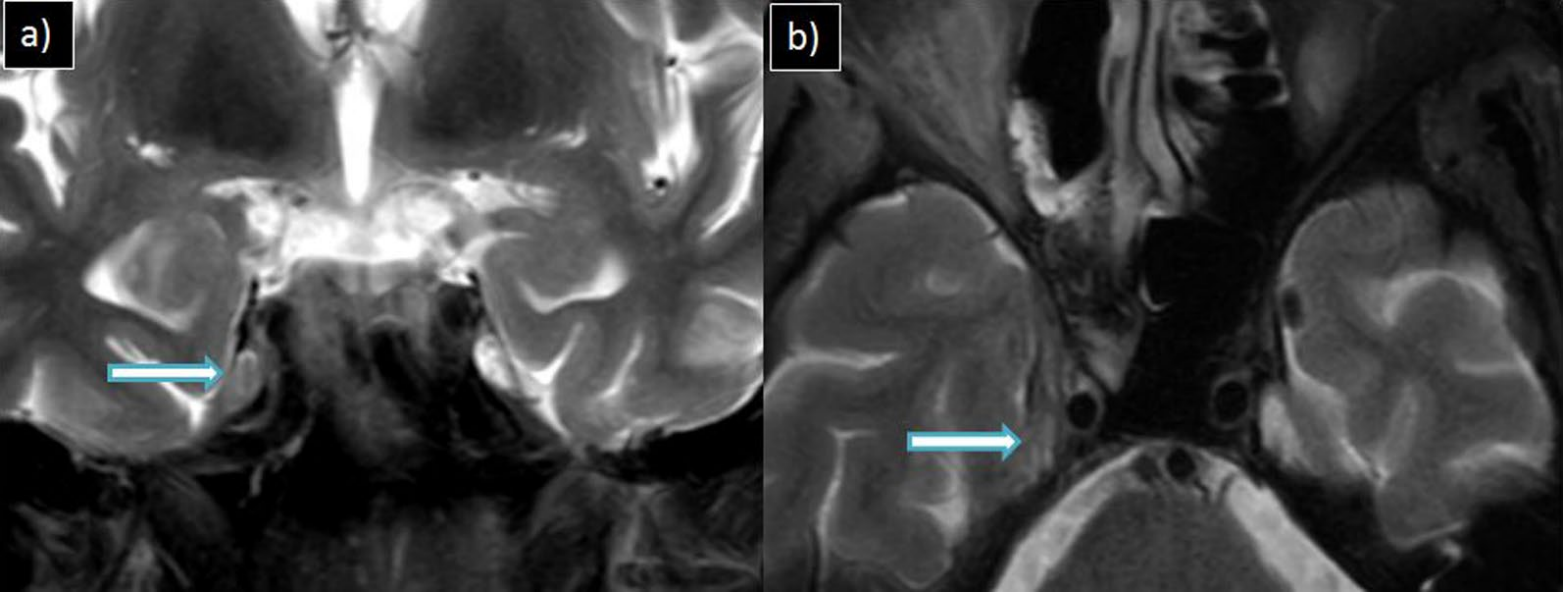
a MRI coronal and b axial T2W-FS images of a 43-year-old male patient with complaints of rhinorrhea, ocular pain and blurring of vision showing altered signal intensity involving the right Meckel’s cave (arrow)
